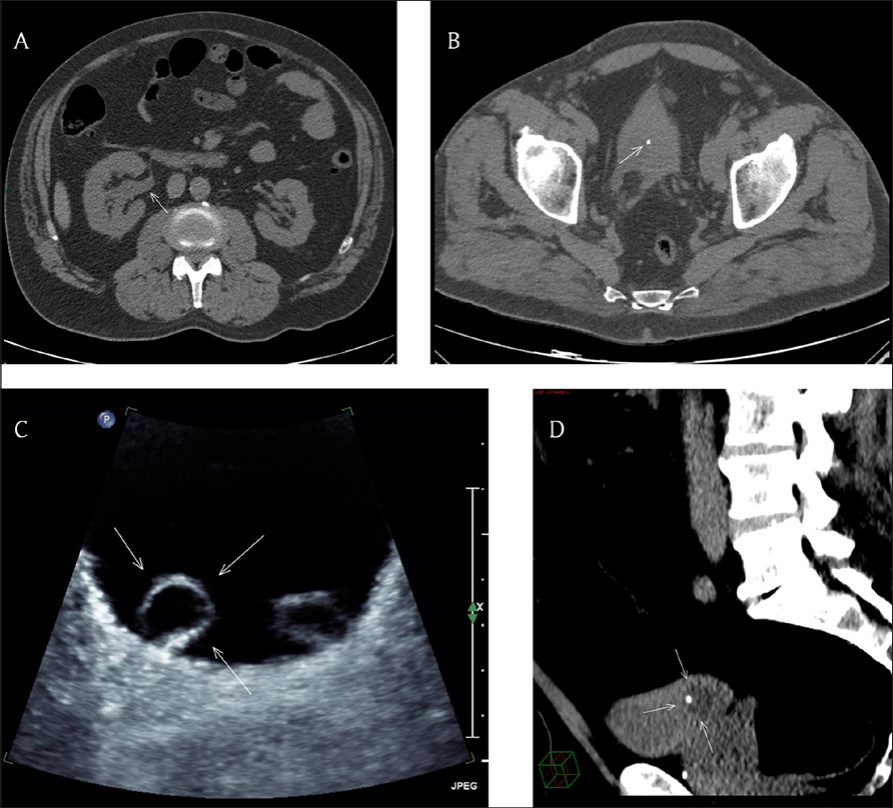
(A) Axial CT scan image (non-contrast) at the level of the abdomen showing right uretero-pyelo-calyceal dilatation (arrow). (B) Axial CT scan image (non-contrast) at the level of the pelvis showing a 3.6 mm hyperdense stone in an almost empty bladder (arrow). (C) Axial US image of the bladder showing bilateral ureterocele, more prominent at right (arrows). (D) Reformatted sagittal CT scan (non-contrast) image with narrow window width of the pelvis showing the urine filled right ureterocele with the stone located inside (arrows).
